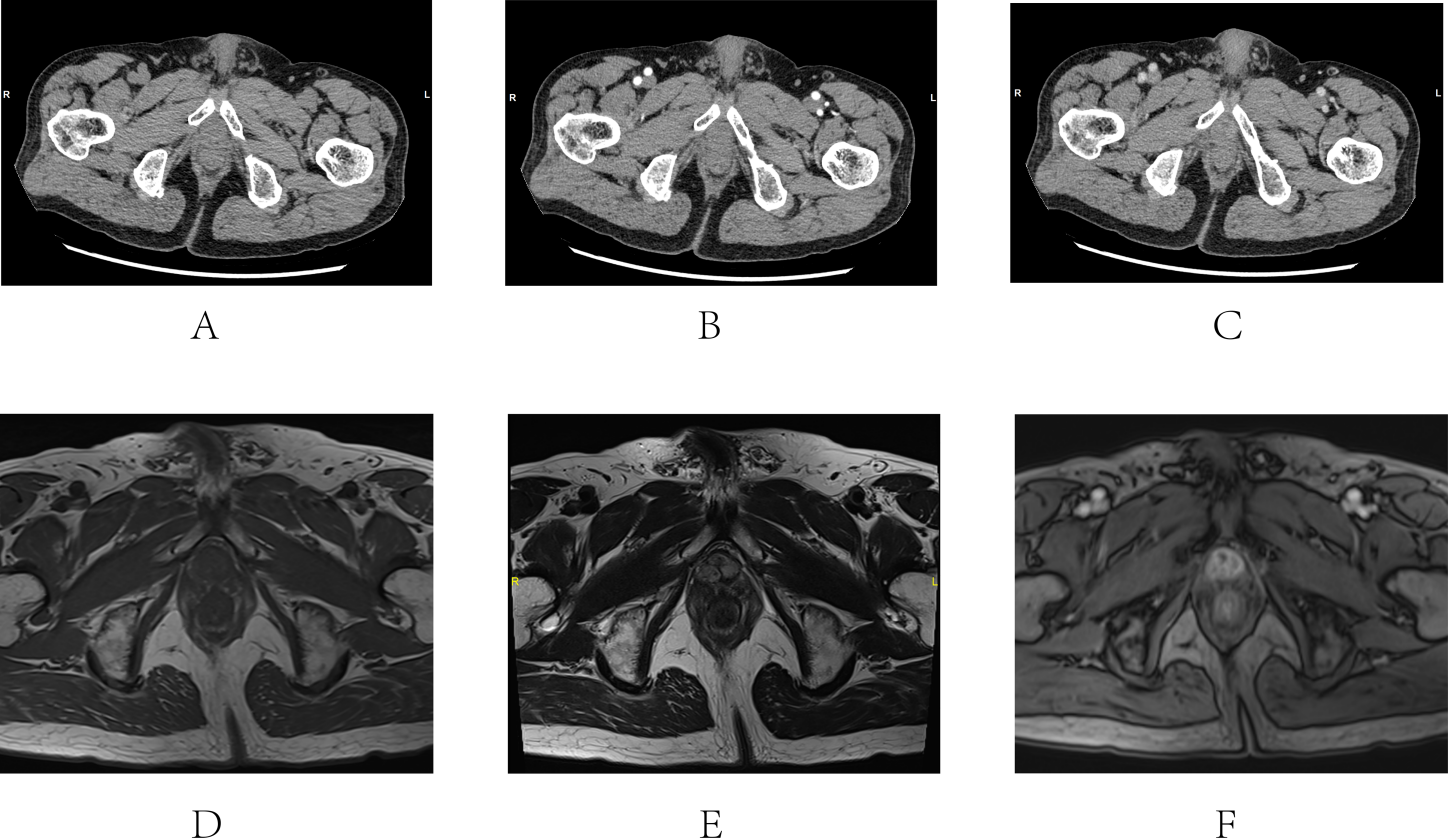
CT and MRI images of Case 2. CT (A-C) An uneven thickening of the prostatic urethra near the bladder neck with a slight enhancement. MRI (D-F) 1.4* 0.9cm nodule was seen at the urethral orifice with a long T2 signal and circular enhancement. (A) Plain scan of the mass; (B) Enhanced scan arterial phase; (C) Enhanced scan venous phase; (D) T1 stage of MRI; (E) T2 stage of MRI; (F) Enhanced T1 stage of MRI.
